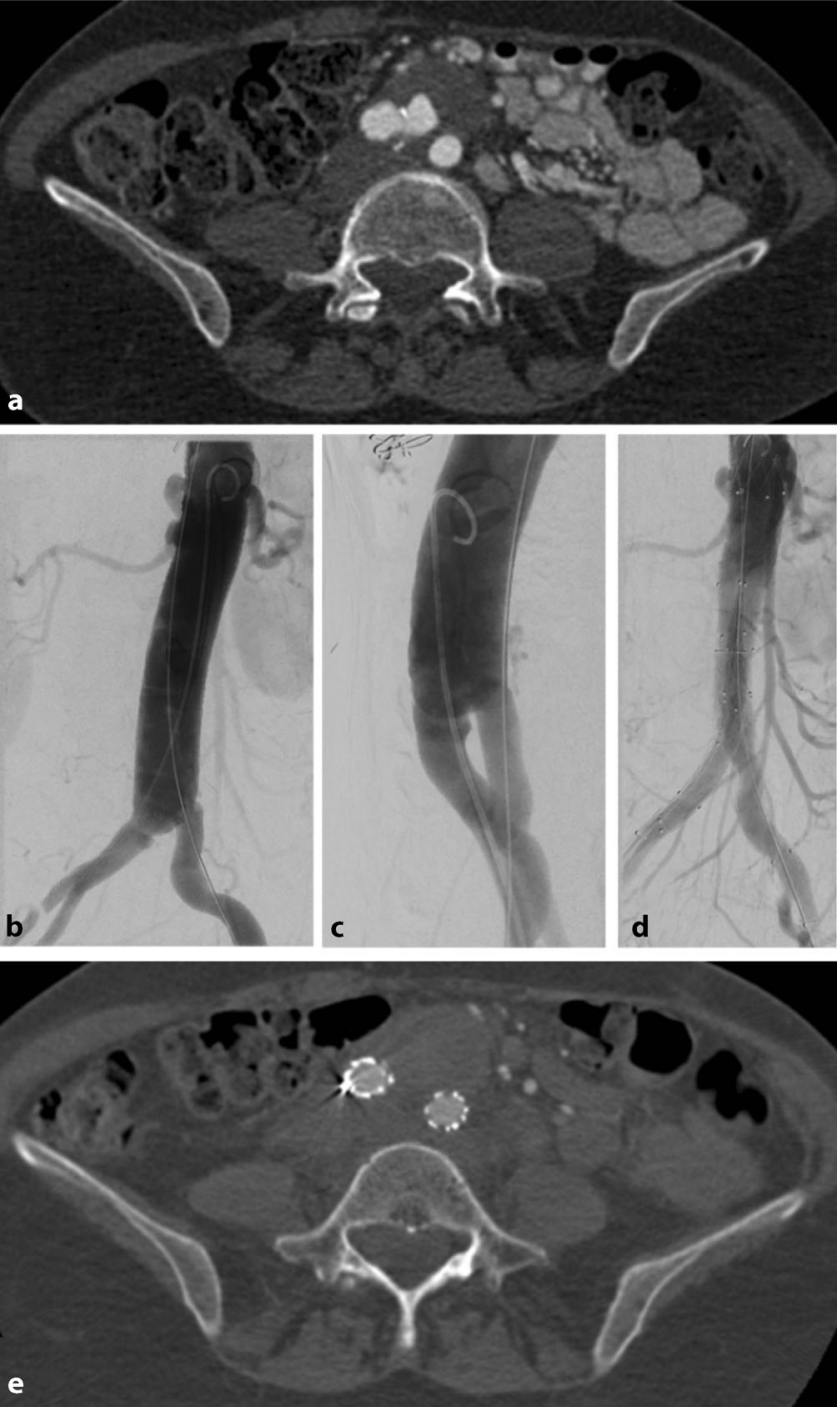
A 48-year-old female patient with Marfan’s syndrome with a contained rupture of a distal anastomotic aneurysm following open thoracoabdominal graft repair. a Preoperative CTA shows a distal connecting aneurysm in the region of the aortic bifurcation, status post-aorto-aortic tube interposition using a Coselli graft. b–c Intraoperative imaging prior to EVAR, anteroposterior and left anterior oblique projections. d Control angiography following successful EVAR showing no indication of an endoleak. e Postoperative CTA showing complete occlusion of the connecting aneurysm. Images kindly provided by the Department of Diagnostic and Interventional Radiology, Heidelberg University Hospital
